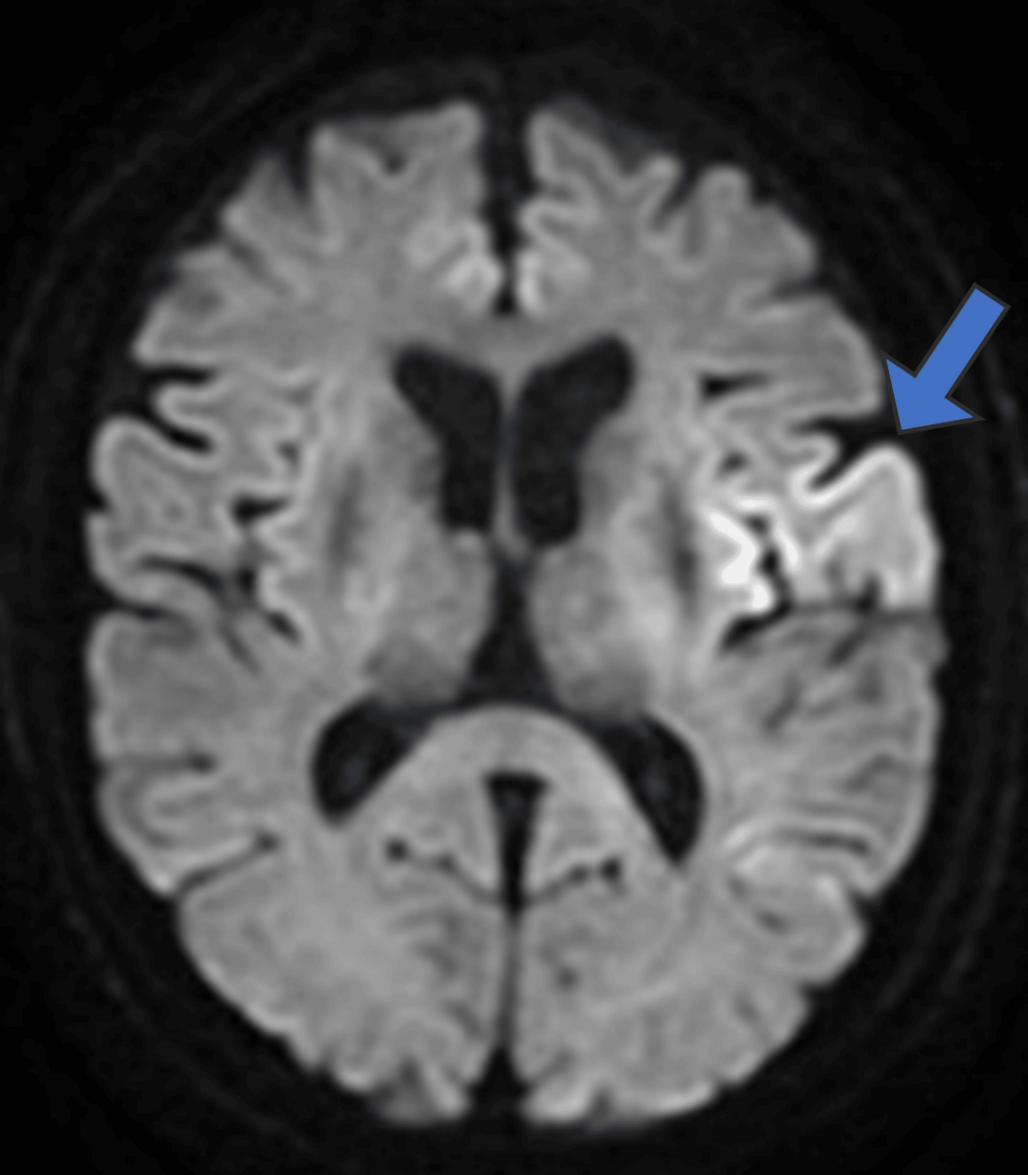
Brain magnetic resonance imaging on postoperative day 1 An axial view of diffusion-weighted imaging demonstrating a high signal intensity in the left frontal lobe. The lesion spans the territories of both the anterior and the middle cerebral arteries, of which findings are consistent with an acute cerebral infarction (arrow).
